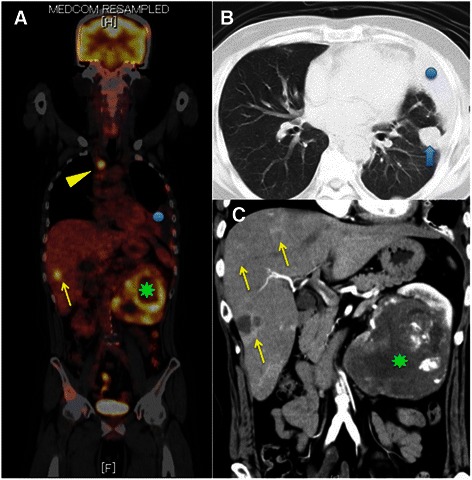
PET/CT scan. A case (50 y.o, male) with pT3aN0M1 (HEP, PUL, OSS) with clear cell carcinoma with sarcomatoid differentiation (Fuhrman grade 4). A: PET scan shows accumulation of FDG in left renal tumor (green asteroid, SUVmax: 14.5), liver (yellow arrows, SUVmax: 7.1), lung (not shown, SUVmax: 3.7), and Th3 vertebra (yellow arrowhead, SUVmax: 8.8). In this case, the highest SUVmax of the metastatic tumors (m-SUVmax) is 8.8. Blue circle shows left pleural effusion. B: Chest CT shows a tumor in left lung (blue arrow) and pleural effusion (blue circle). C: Enhanced abdominal CT shows large left renal tumors (green asteroid) and multiple liver tumors (yellow arrows).
